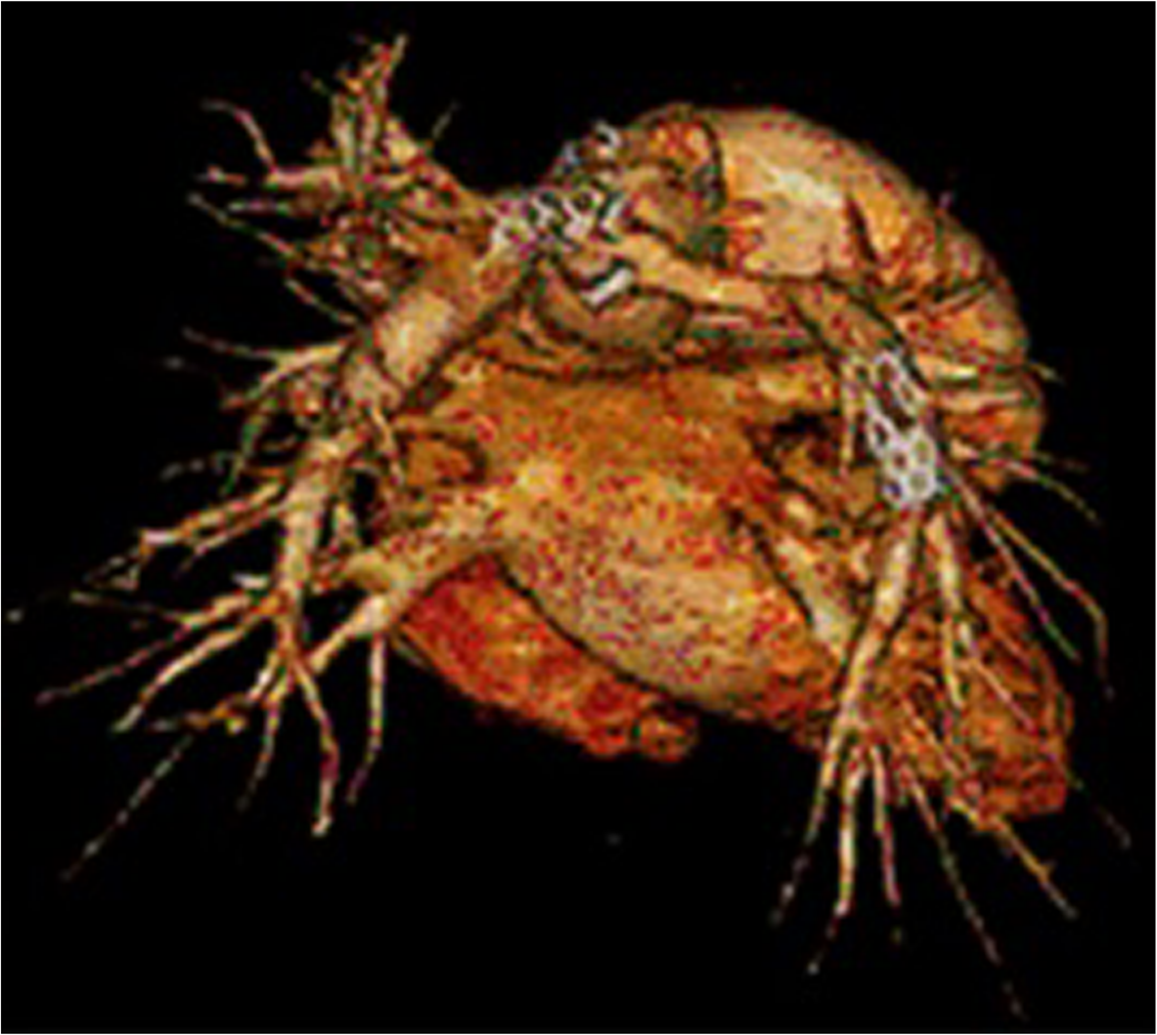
CT Angiography 3 years after the total repair by Contegra graft between the right ventricle and the innominate vein. The distal Contegra graft stenosis was stented. Please note the migration of the second stent from the left pulmonary artery into the distal left lung hilum
